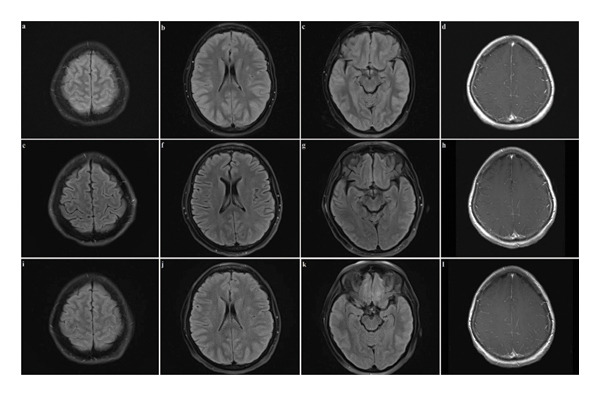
Magnetic resonance imaging (MRI) scan of the axial images of the brain. (a), (b), (c) T2 fluid‐attenuated inversion recovery (FLAIR) images acquired on Day 4 of initial presentation, showing diffuse gyral edema, sulcal and ventricular effacement, and sulcal hyperintensities. (d) Contrast‐enhanced T1 image acquired on Day 4, showing slightly increased sulcal enhancement. (e), (f), (g) T2 FLAIR images acquired on Day 19, showing resolution of the findings at Day 4. (h) Contrast‐enhanced T1 image acquired on Day 19, showing resolution of findings at Day 4. (i), (j), (k) T2 FLAIR images acquired upon repeat presentation, showing recurrence of the abnormalities found on Day 4 of initial presentation. (l) Contrast‐enhanced T1 image acquired upon repeat presentation, showing recurrence of mild sulcal enhancement.
